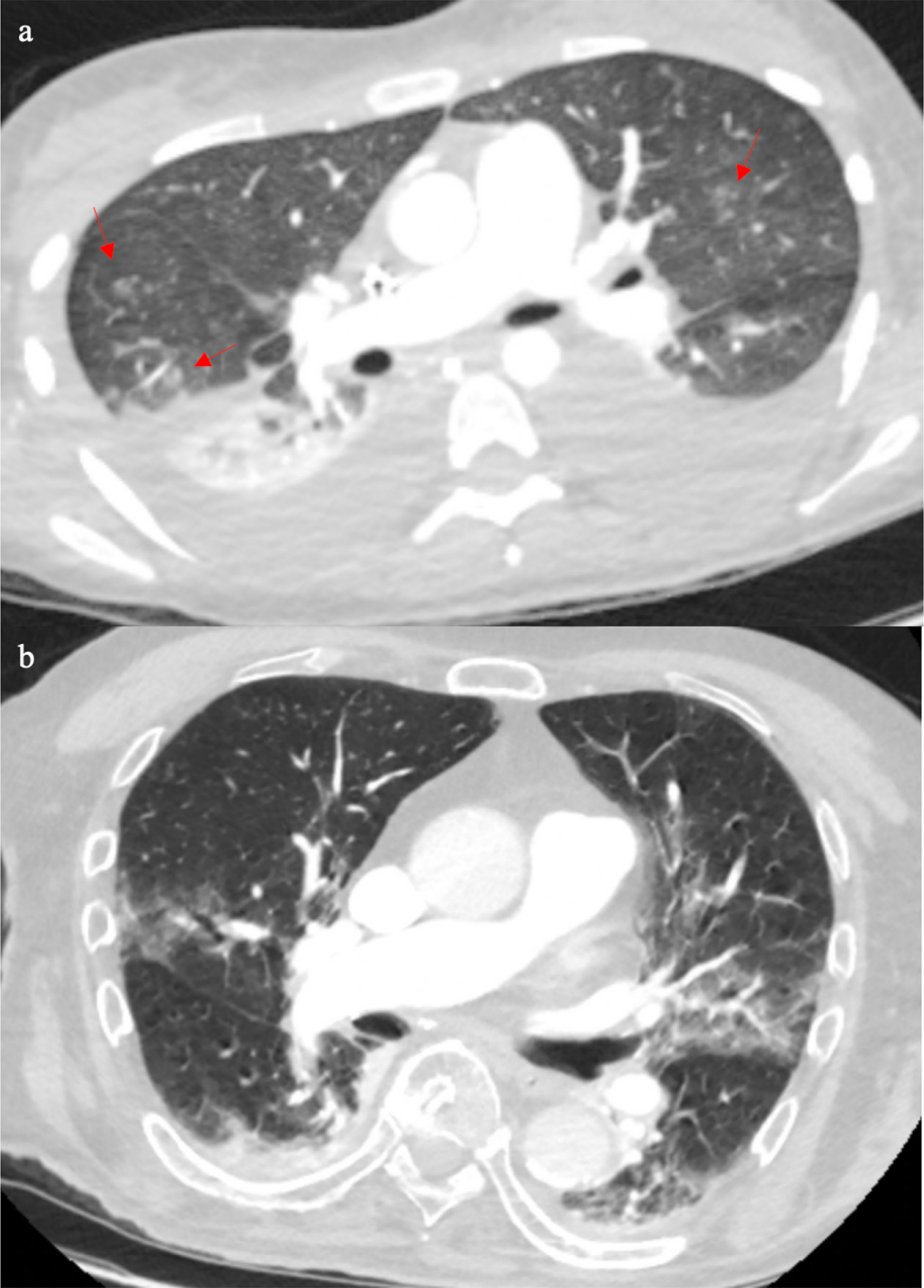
Patient D’s CT (4a) showed bilateral pleural effusions with patchy ground glass and ground glass nodularity (arrows), which is more common in bacterial infections, or if someone is immunocompromised, fungal infection is also a possibility. Patient E’s CT (4b) showed bilateral small pleural effusions with bronchocentric ground glass opacification, which in the right clinical context it could be supportive of COVID-19 pneumonia.
